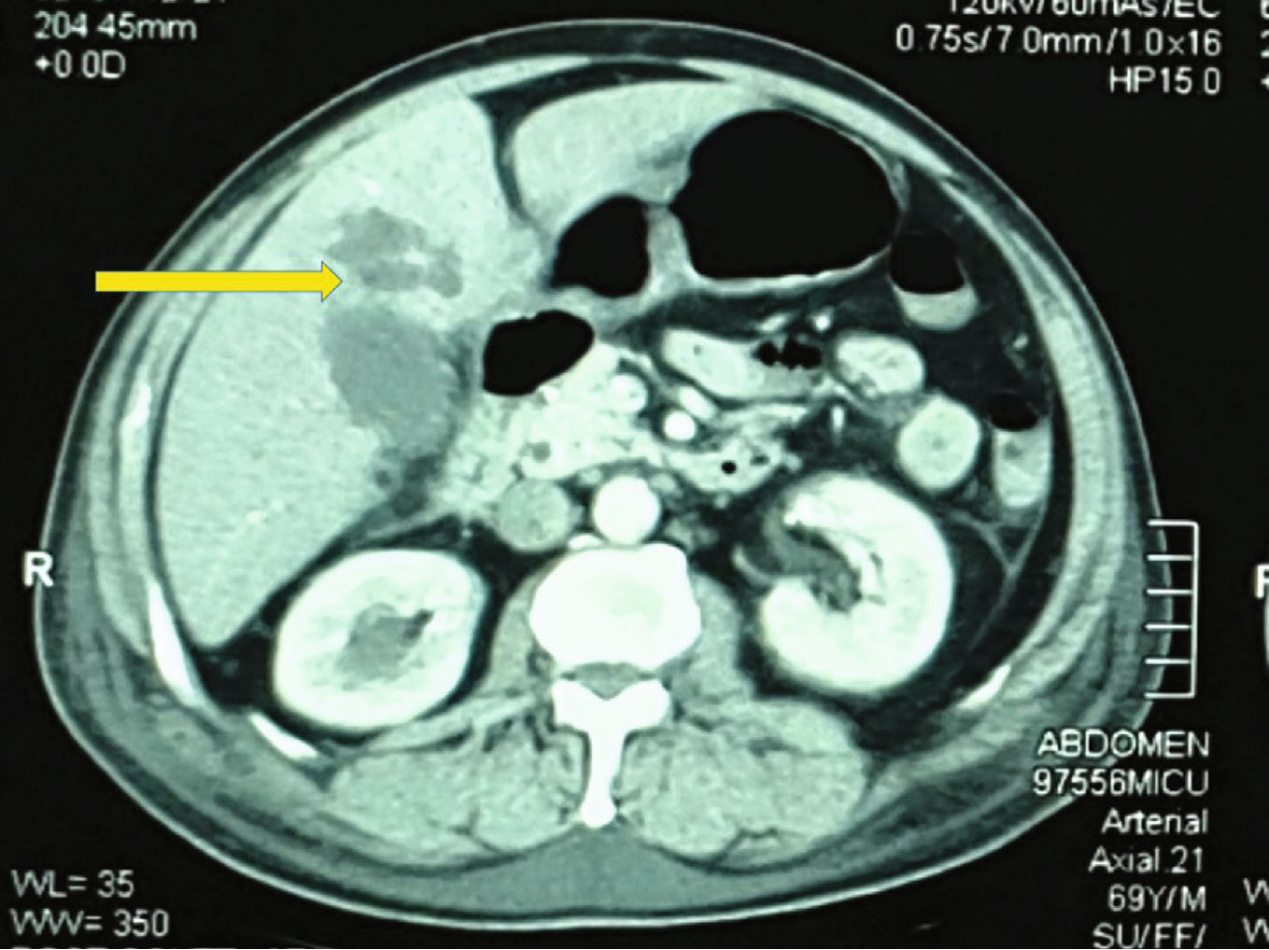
Contrast-enhanced computed tomography of the abdomen showing a 3 × 3 × 2 cm ill-defined, nonenhancing, hypodense area in segment V of the liver, compatible with a liver abscess.
